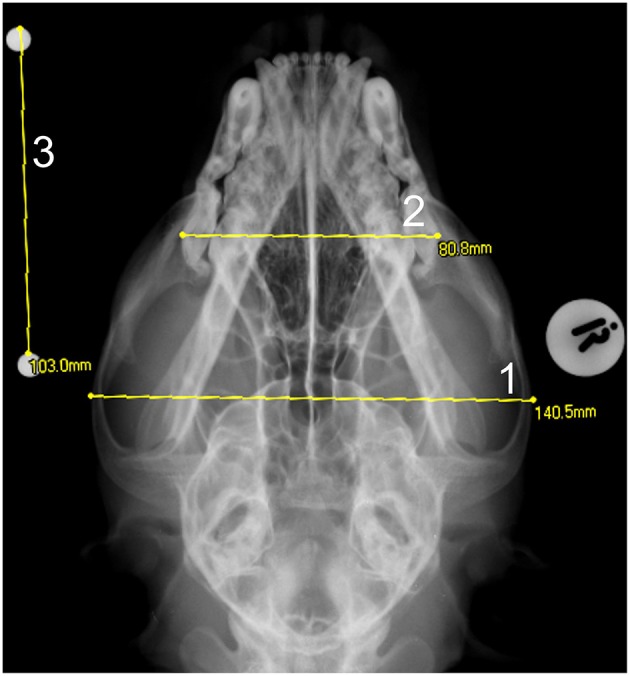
A dorso-ventral skull radiograph of a cheetah cub showing the measurement of the: 1- maximum skull width and 2- the maximum maxilla width. A 100 mm scale bar was placed at a height equal to the middle of the skull in order to quantify the enlargement factor -3.
